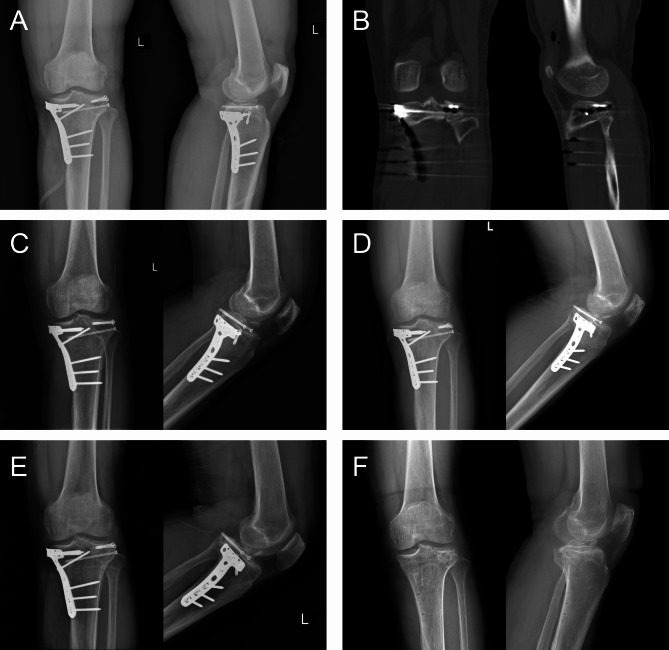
The patient’s radiographic images after the operation. (A) The anteroposterior and lateral X-ray after operation immediately. (B) The coronal and sagittal CT images after operation immediately. C, D, E. The anteroposterior and lateral X-ray at 1 month, 3 months and 12 months after operation. F. The anteroposterior and lateral X-ray at 15 months after operation (after internal fixation removal)
